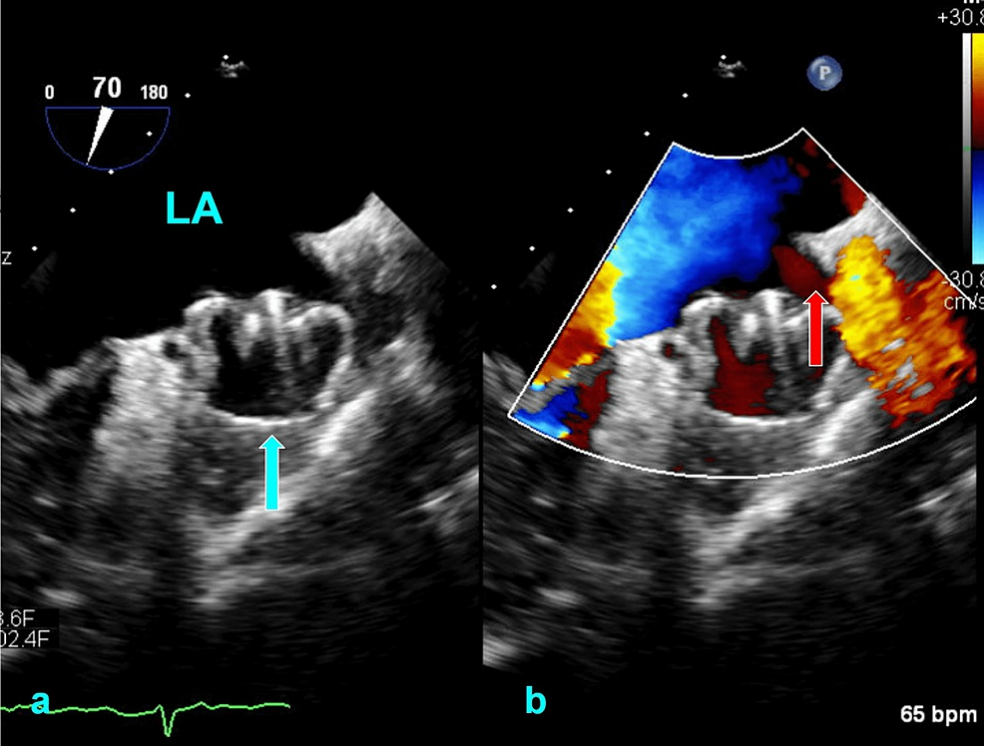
Right ventricle inflow-outflow view on the transesophageal echocardiogram (TEE) shows evidence of left atrial appendage occlusion (LAAO) failure at 10 months post-procedure. (a) TEE without color Doppler shows the Watchman device (blue arrow) seated in the left atrial appendage (LAA). (b) TEE with color Doppler shows evidence of flow around the Watchman device, indicating a peri-device leak (red arrow). LA: left atrium
